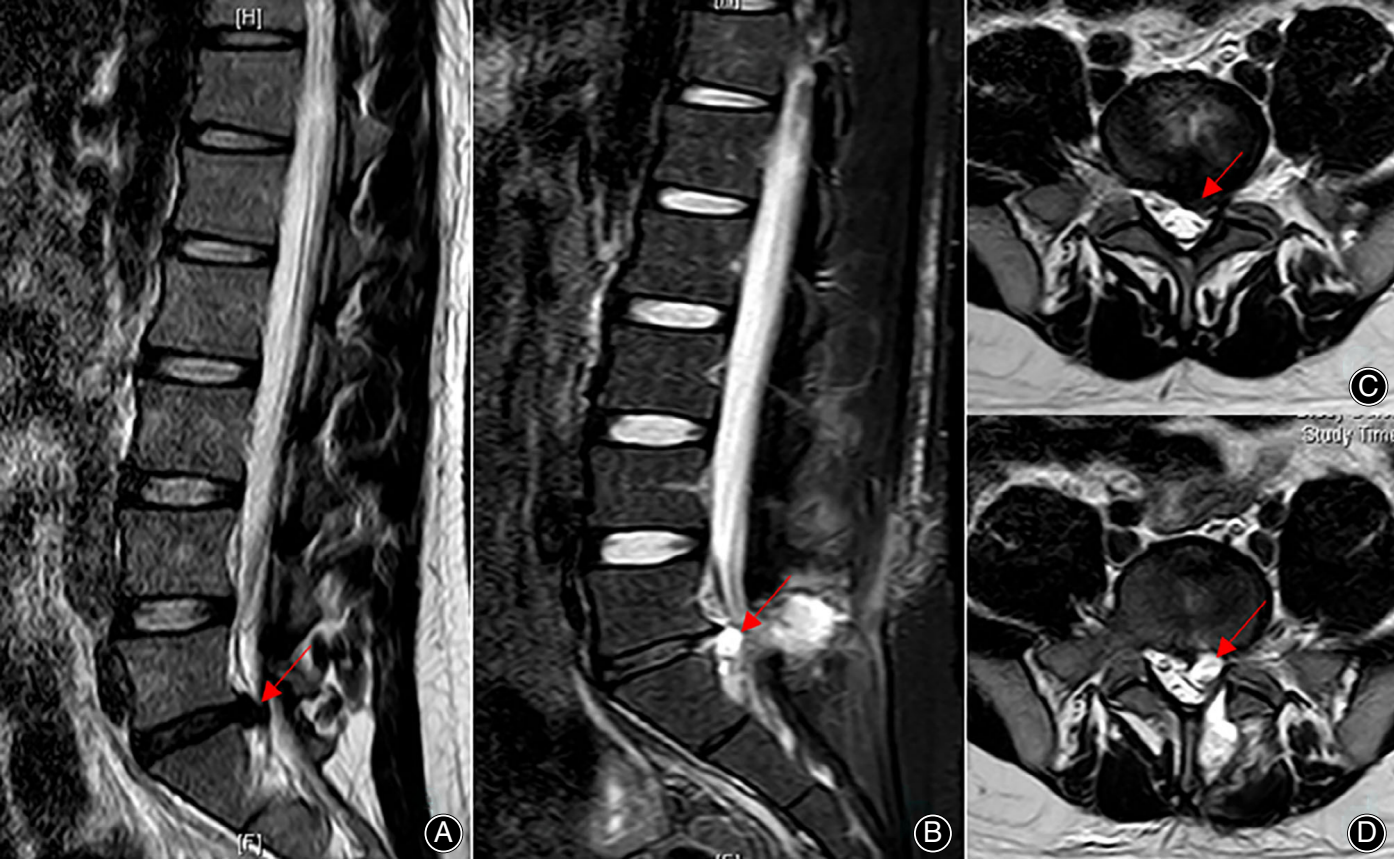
Lumbar MRI: disc degeneration (Pfirrmann Grade IV) and PDP. A\C: disc degeneration before lumbar discectomy, B\D: PDP (L5/S1 left).
